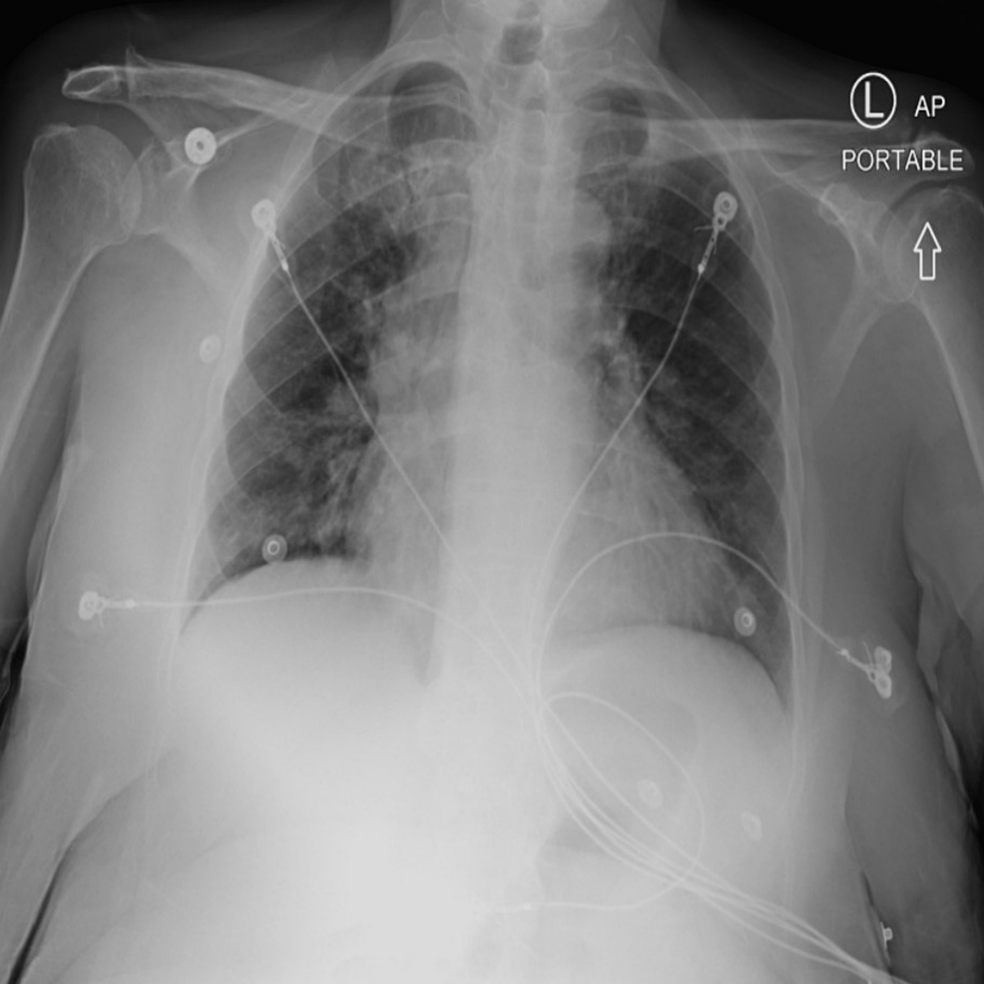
Chest X-ray showing moderately enlarged cardiac silhouette and mild pulmonary vascular congestion.
